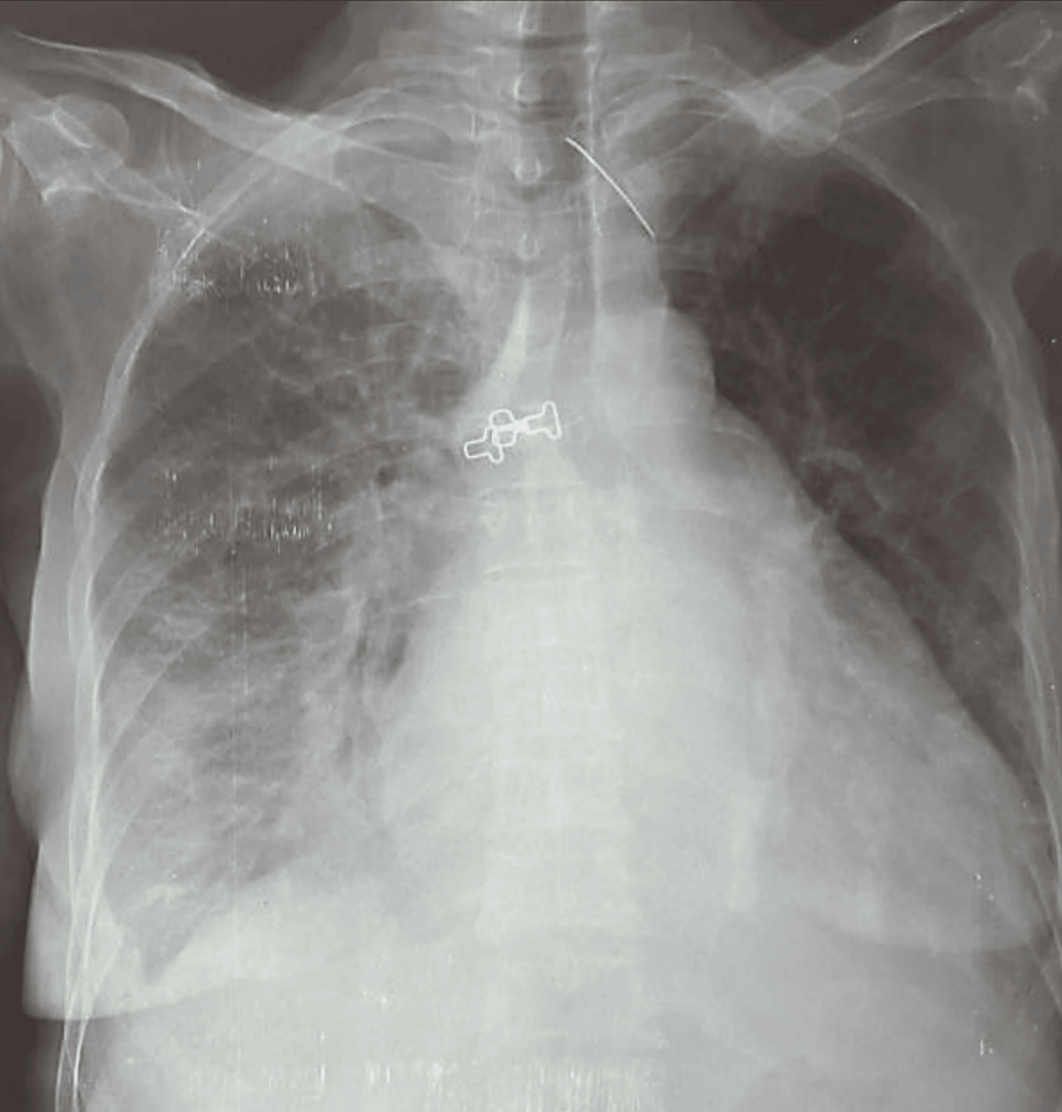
Chest X-ray showing a mitral configuration of the cardiac silhouette: cardiomegaly with a supradiaphragmatic apex, convexity of the mid-left cardiac border, and a double contour (double density) of the right lower cardiac border. Chest X-ray of a 45-year-old woman presenting with shortness of breath and palpitations.
